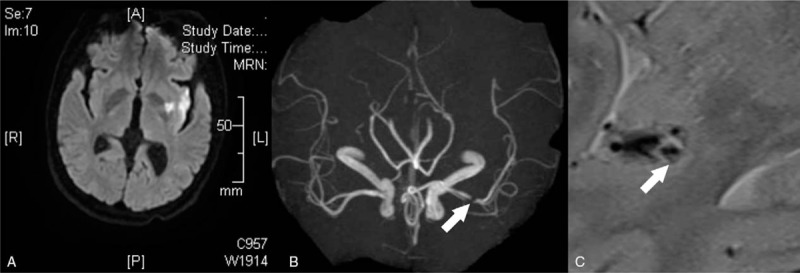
Brain diffusion-weighted magnetic resonance imaging revealed focal hyperintensities in the left insula and basal ganglia (A). Head magnetic resonance angiography showed an angiostenosis (arrow) in the initial part of the middle cerebral artery (B). MRI of the arterial walls revealed a double-lumen sign (C, arrow).
